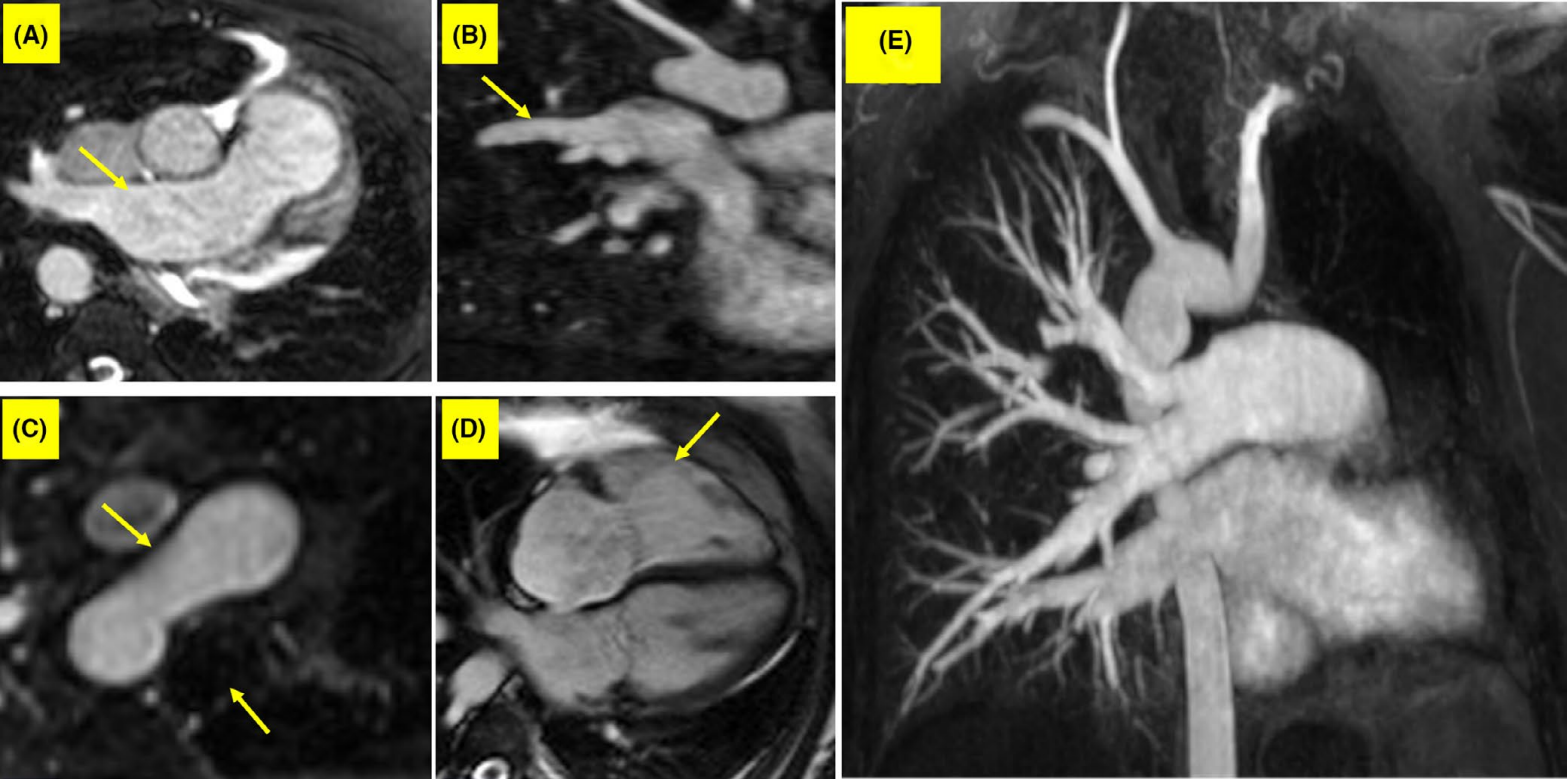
Cardiac magnetic resonance images of the patient with UAPA show (A) absence of LPA with RPA dilation (arrow), (B) PAPVC between RUPV and SVC (arrow), (C) right‐sided aortic arch (upper arrow, with the lower arrow pointing to the trachea), (D) mild RV enlargement (arrow) in the 4‐chamber view, and (E) diminished left lung vascular markings. Abbreviations: CMR, cardiac magnetic resonance; UAPA, unilateral absence of pulmonary artery; LPA, left pulmonary artery; RPA, right pulmonary artery; PAPVC, partial anomalous pulmonary venous return; SVC, superior vena cava; RV, right ventricle
